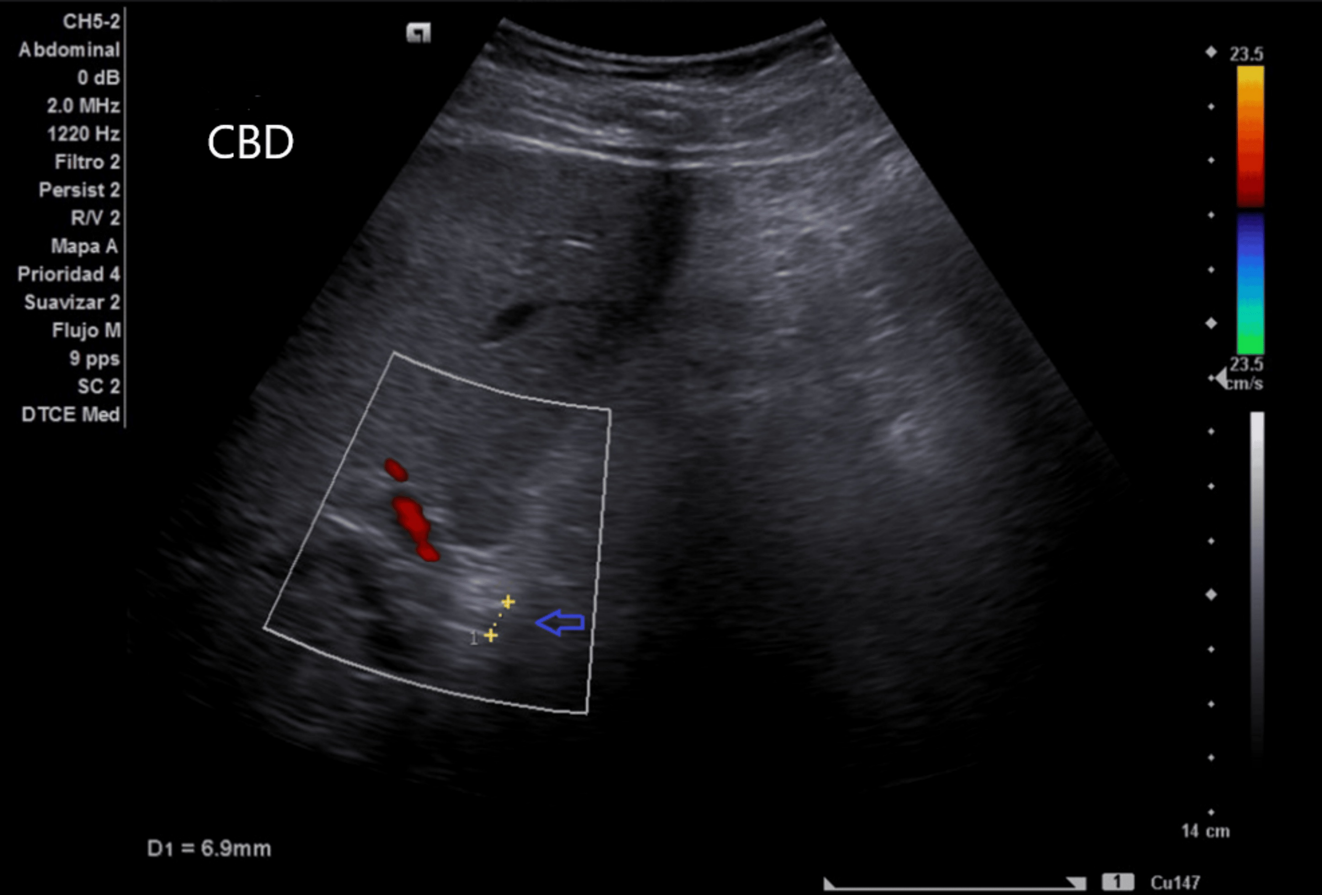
Initial ultrasonography Ultrasound demonstrating CBD dilation up to 1 cm (blue arrow). CBD: common bile duct
